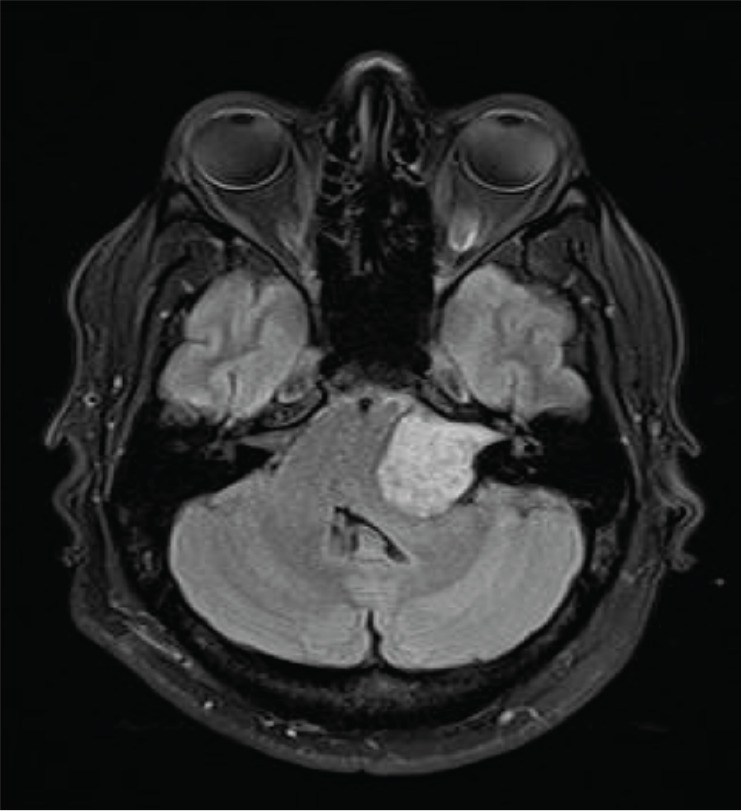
Brain MRI revealed a mass located in the posterior fossa within the cisterna of the left pontocerebellar angle.
